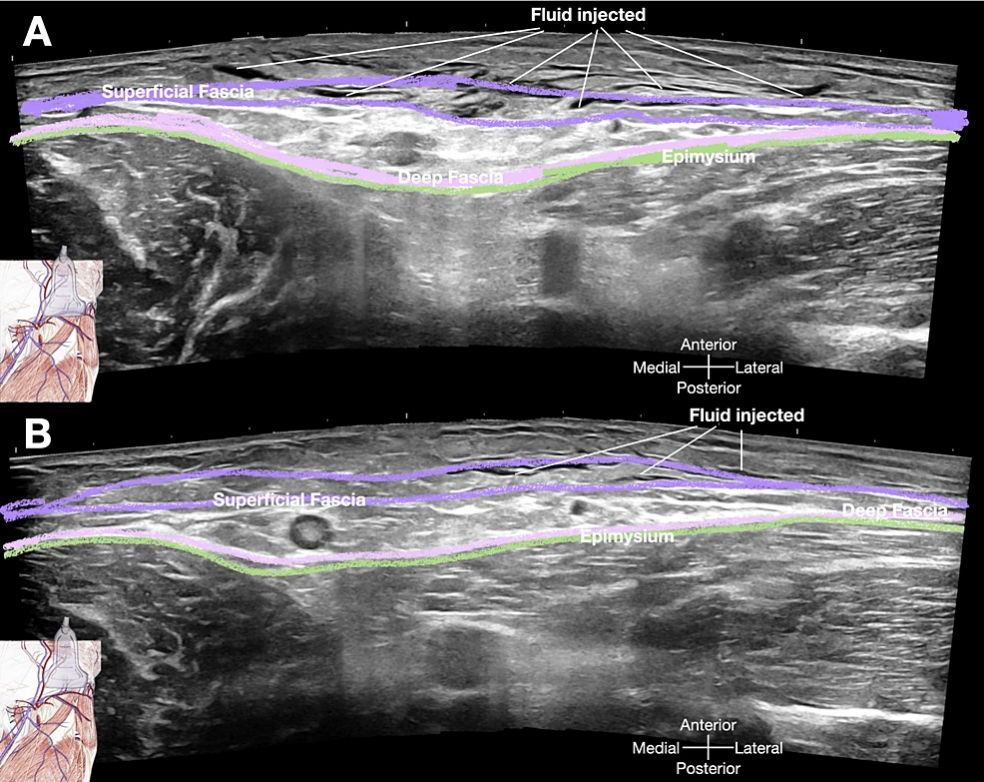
Post-ultrasound-guided hydrorelease/hydrodissection ultrasound findings A: This image, captured 30 seconds after the injection, demonstrates the immediate dispersal of the injected solution within the superficial fascia and surrounding soft tissues. The solution appears as a diffuse, hypoechoic/ anechoic area within the previously hyperechoic fascial plane, indicating successful separation and relaxation of the fascial layers. Additionally, the underlying structures, such as the adductor muscle group and the femoral neurovascular bundle, are clearly visualized, confirming the absence of inadvertent spread of the solution to these critical areas. B: This image, obtained two minutes post-injection, shows further spreading of the injectate. The fascial plane appears less echogenic compared to the pre-injection state. Image credit: Dr. Lam K
